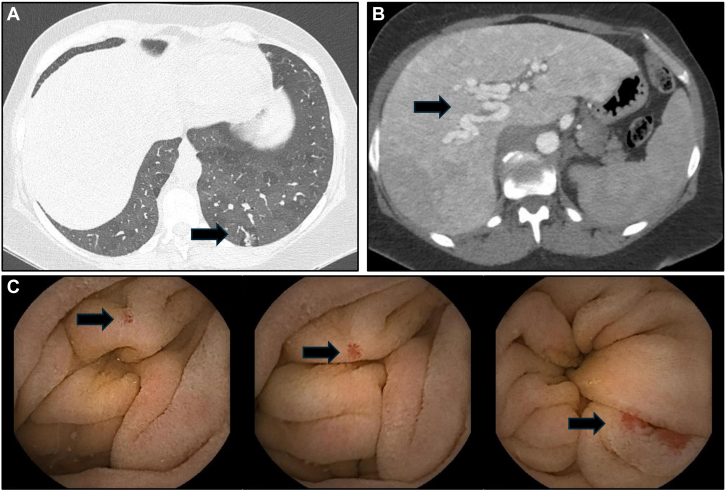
A-C, Arrows indicate (A) arteriovenous malformation in the chest CT scan in left lower lobe; (B) abdomen CT scan with multiple hepatic arteriovenous malformations: the largest 3.5 cm, and (C) multiple gastrointestinal telangiectasias during endoscopy.
